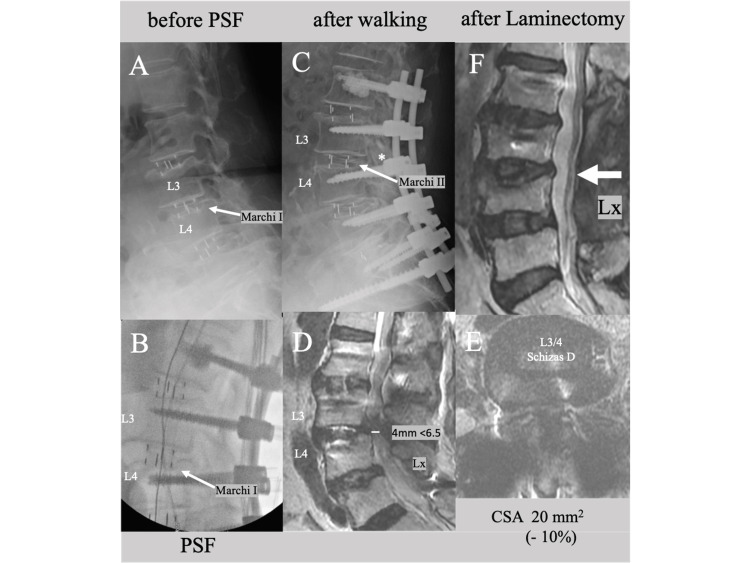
Lateral lumbar X-rays nnd MRI images after OLIF, PSF and an additional laminectomy of Case 3 A lateral lumbar X-ray (A) taken shortly before the second posterior operation (following a fall) showing mild endplate injury and cage subsidence at L3/4 (Marchi grade I, arrow). A lateral lumbar fluoroscopic image during pedicle screw fixation (B) showing no deterioration of cage subsidence. After commencing walking, a lateral lumbar X-ray (C) showing advancement of the cage subsidence (Marchi grade II, arrow), with a decrease in PDH (asterisk) at L3/4. MRI images showing (D) a decrease in the anteroposterior diameter (APD) of the dural sac (from 5 mm to 4 mm) and (E) a 10% decrease in CSA. After performing an additional laminectomy at L3/4, an MRI image (F) showing sufficient enlargement of the dural sac. PSF: posterior screw fixation, PDH: posterior disc height, CSA: cross-sectional spinal canal canal area
